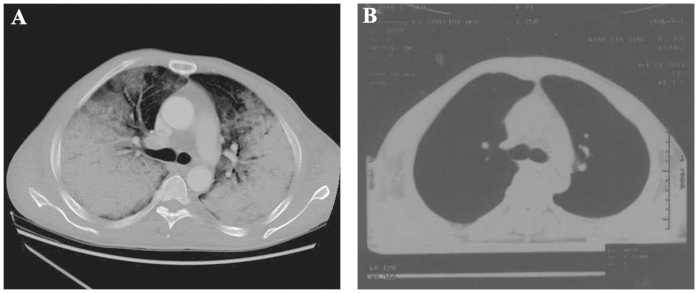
Transaxial computed tomography images of the chest showing infiltration of the lungs (A) prior to chemotherapy. The bilateral lungs had a large area with a dark appearance, and mediastinal lymph nodes were visible.(B) Transaxial computed tomography images of the chest following chemotherapy.
